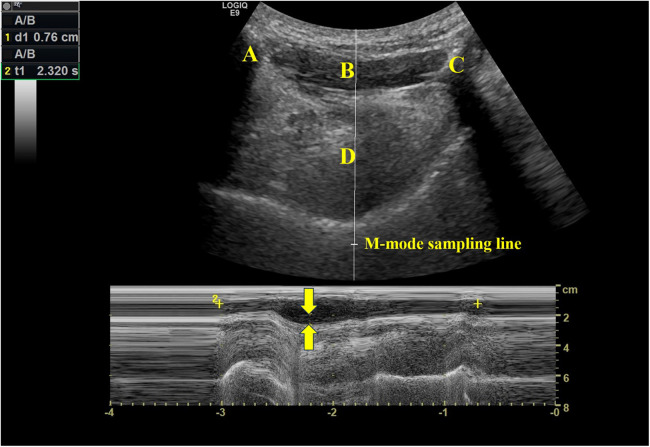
Ultrasound image with labeled anatomical areas A, B, C, and D, and an M-mode sampling line intersecting the regions. The lower section displays a corresponding M-mode tracing, with yellow arrows indicating measurement points. Measurement data include a distance of 0.76 cm and a time of 2.32 s.
